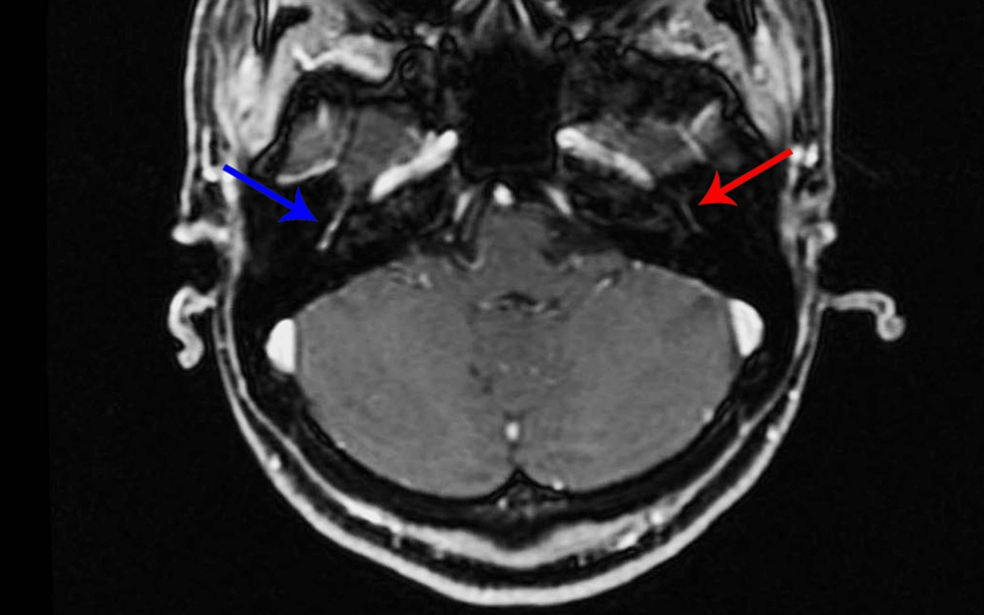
Contrast-enhanced axial MRI performed in February 2022 demonstrates abnormal enhancement of the right facial nerve (blue arrow). The left facial nerve (red arrow) is normal.
